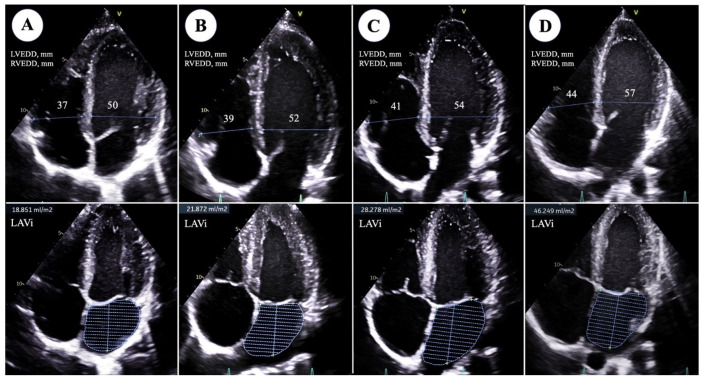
Echocardiographic comparison of main morphological parameters (left ventricle end-diastolic diameter, LVEDD; right ventricle end-diastolic diameter, RVEDD; LAVi: left atrial volume indexed) between athletes practicing different track and field subspecialties: (A) 100–200 mt; (B) 400 mt; (C) 1500 mt; (D) marathon.
